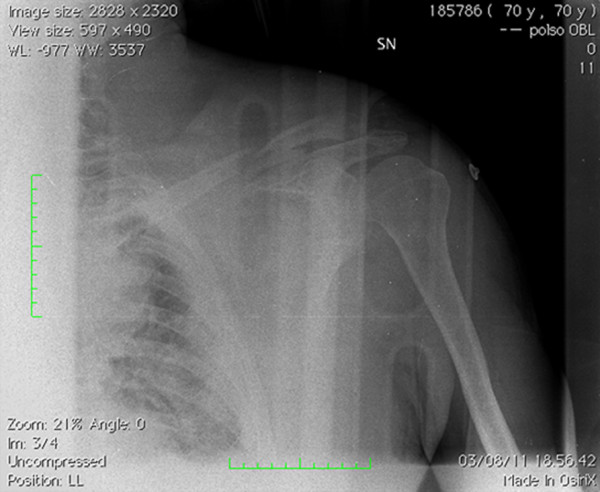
Plain radiography showing left midshaft clavicular fracture.
